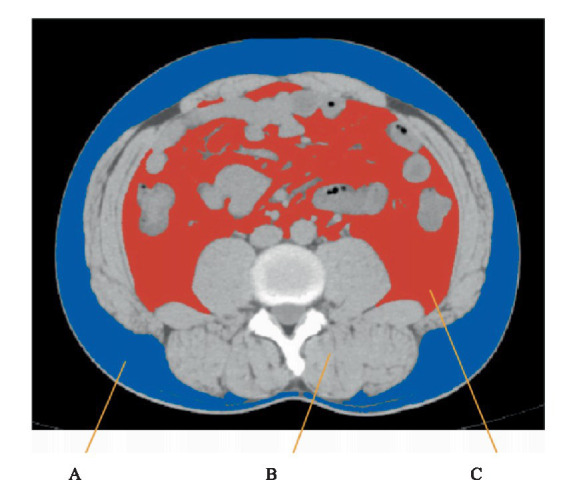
This image selects the abdominal CT scan at the lower edge of L3. A, Abdominal subcutaneous adipose; B, abdominal muscles; C, abdominal visceral fat.
